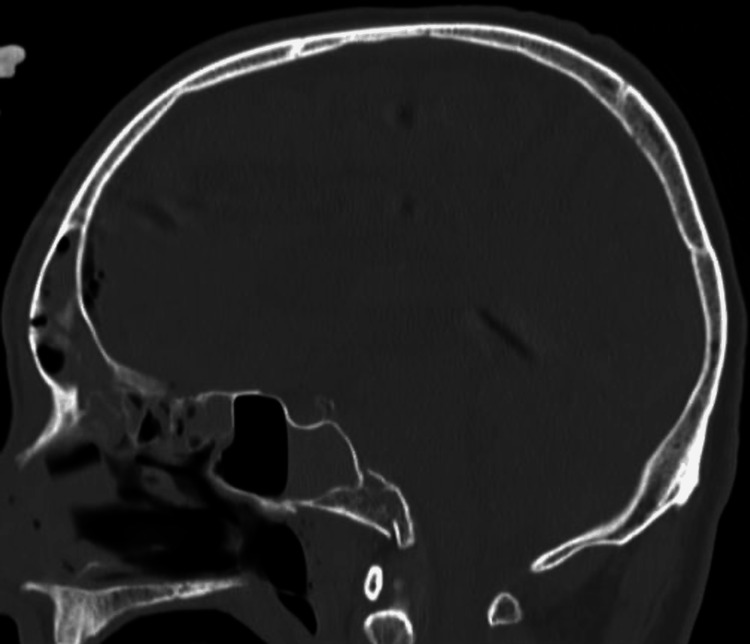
Sagittal-view bone-window computed tomography after reducing fracture of the clivus on hospital day two. Although the entire head was repositioned by about 2 mm, incarceration of the fractured bone fragment was not released.
